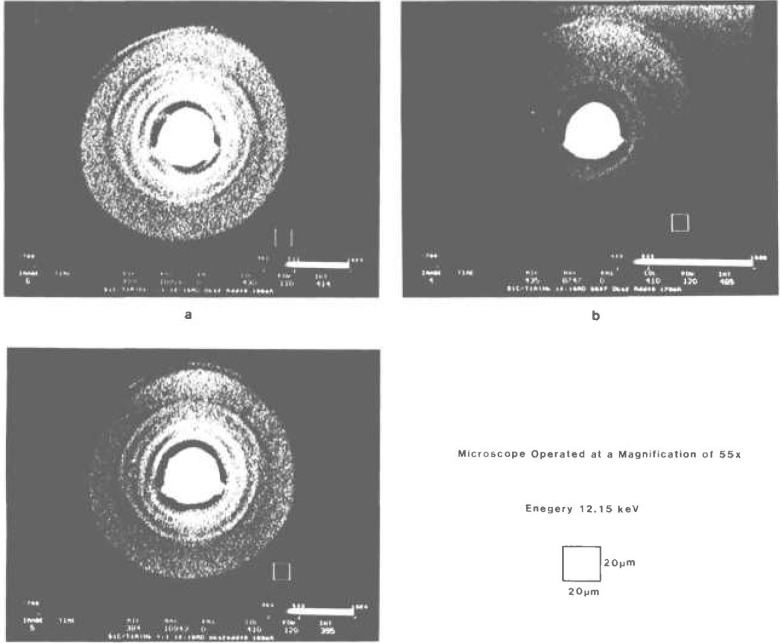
Three views of the SiC fiber at a magnification of 55× and 12.15 keV. The sample is oriented (a) −0.1°, (b) 0.0°, and (c) +0.1° off from the x-ray beam direction.
